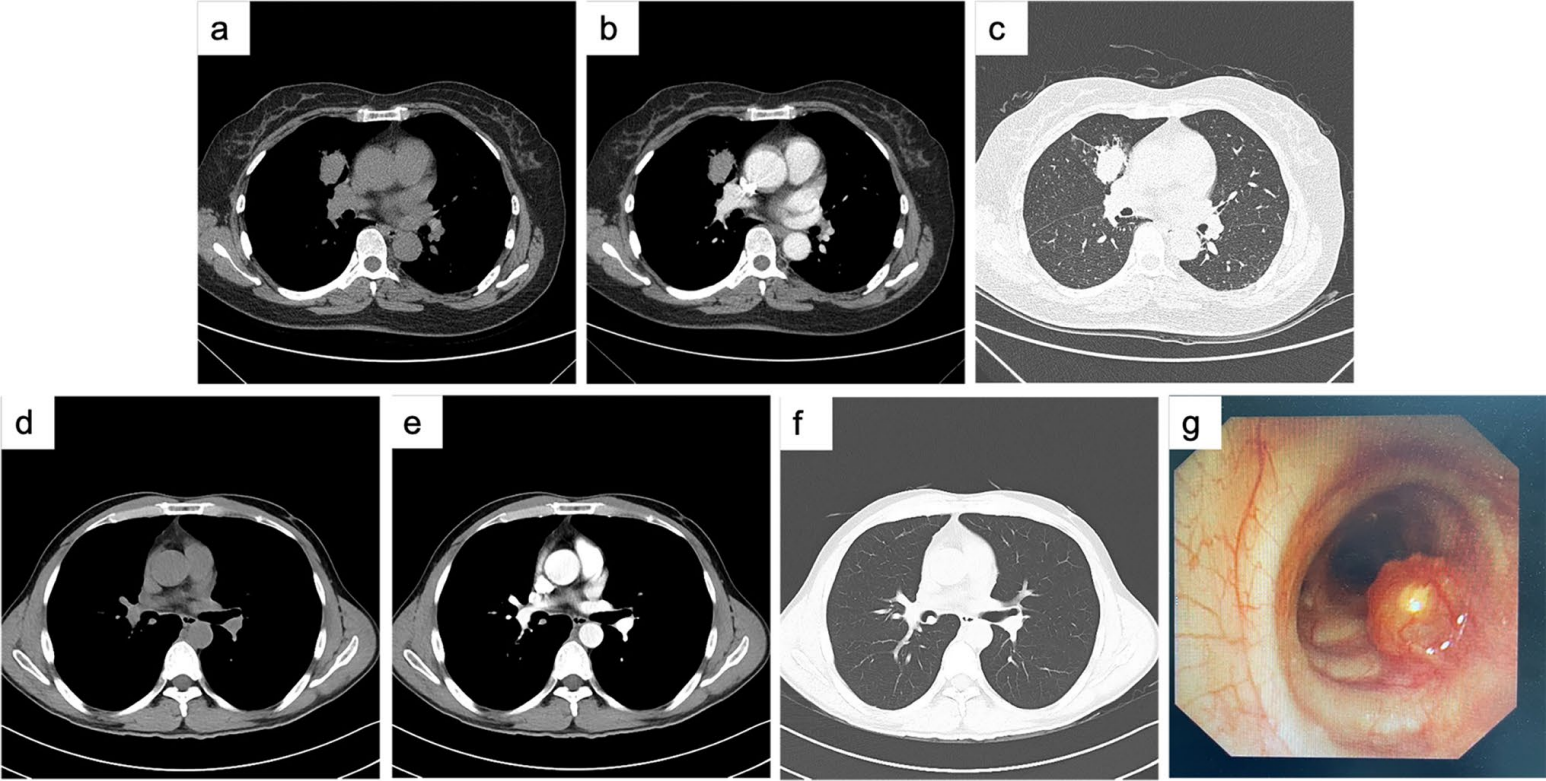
CT and bronchoscopy images of pulmonary mucoepidermoid carcinoma of peripheral type (a–c) and central type (d–g). a, d Plain transverse image of mediastinal window; b, e Enhanced transverse image of mediastinal window; c, f Transverse image of the lung window; g Bronchoscopy images
